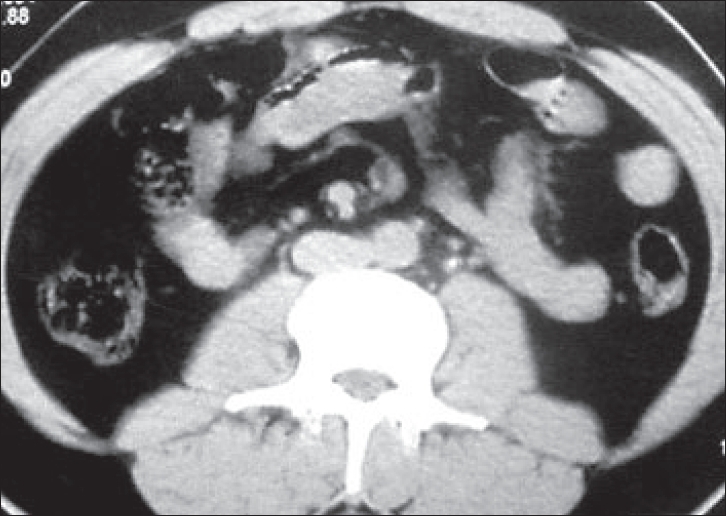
Non-contrast computed tomography showing left ureteric calculus
